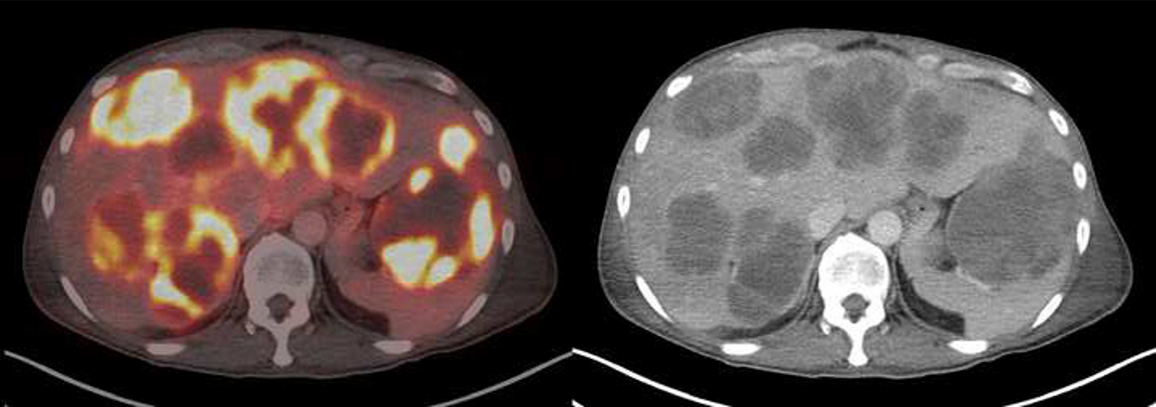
Transaxial PET/CT fusion (left) and CT (right) images showed that the largest splenic mass measured 9.6 × 7.5 cm with maximum standardized uptake value (SUV_max_) 15.8. The liver and splenic lesions were almost entirely necrotic, with a thin rim of intense ^18^F-FDG uptake.
